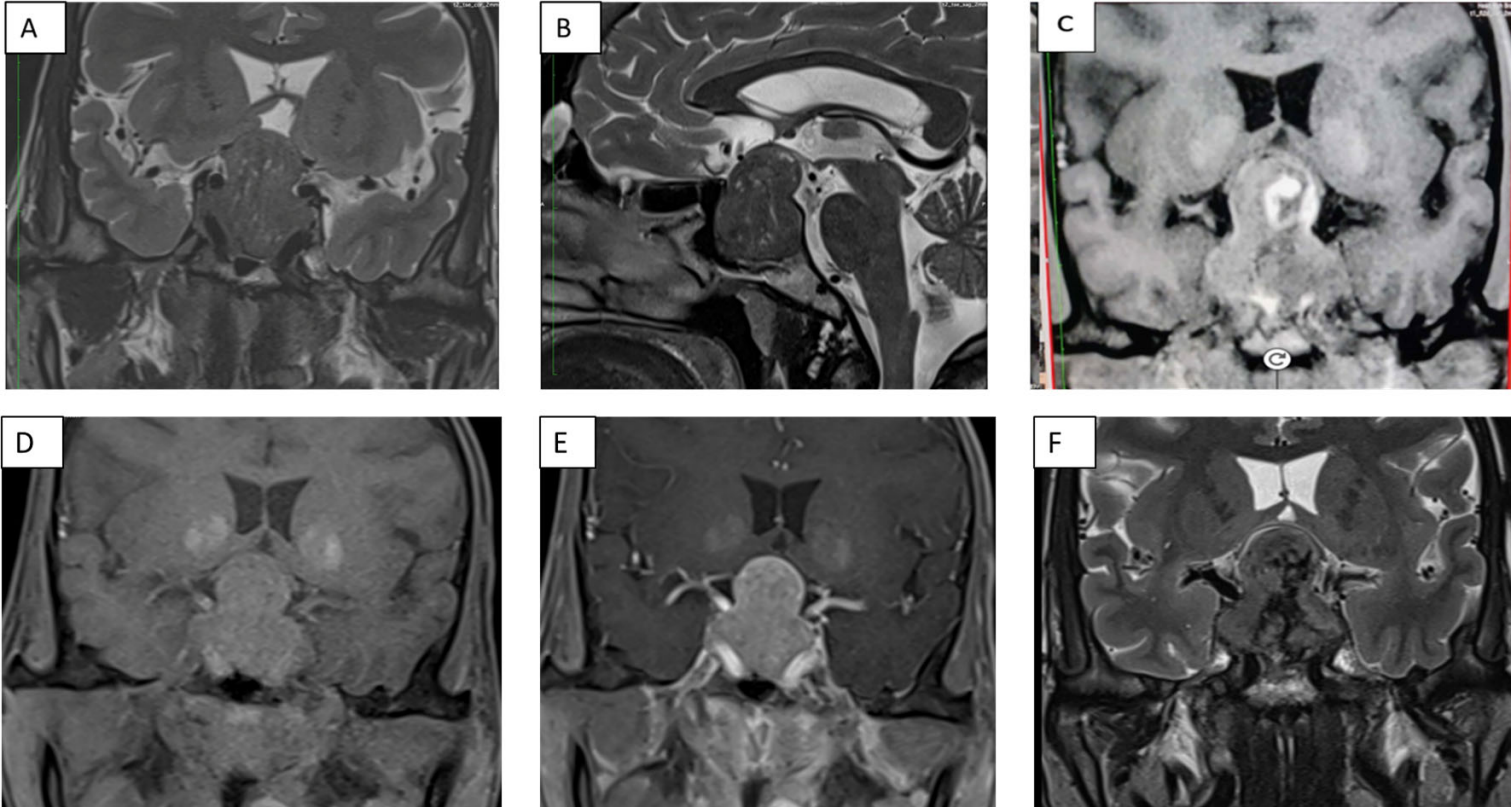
Panel of contrast-enhanced MRI of the sella showing (A) a 22×30×34 mm hypointense heterogenous lesion on a T2-weighted sequence coronal section (A) and sagittal section (B), and coronal T1-weighted non-contrast (D) and post-contrast (E) sections. T1-weighted non-contrast (C) and T2-weighted (F) coronal sections show hyperintensities and hypointensities, respectively, with an increase in size of the lesion to 21×35×41mm, which is suggestive of pituitary apoplexy.
